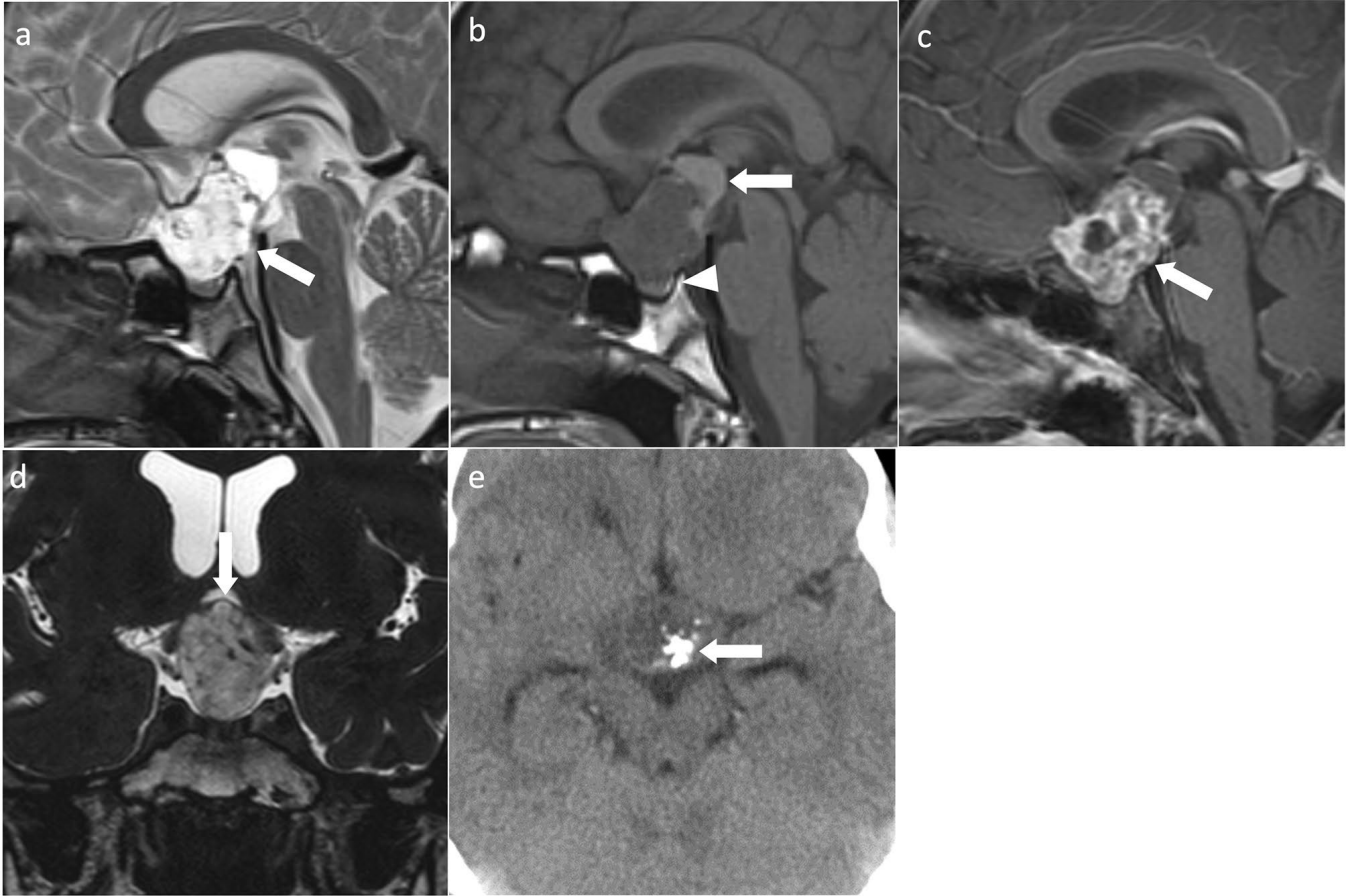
Adamantinomatous craniopharyngioma. A 13-year-old girl with headache and vision loss. a Sagittal T2WI shows a multifocal cystic mass above the sella turcica (arrow). The pituitary gland is observed within the sellar turcica. b Sagittal T1WI shows some cysts with mild hyperintensity (arrow) compared to white matter. Normal hyperintensity in the posterior pituitary gland is observed (arrowhead). c Sagittal contrast-enhanced T1WI shows heterogeneous contrast enhancement. The pituitary stalk is inside the mass and cannot be identified (arrow). d Coronal heavy T2WI shows the optic chiasm compressed by the mass (arrow). e Non-contrast computed tomography shows calcification inside the mass (arrow)
